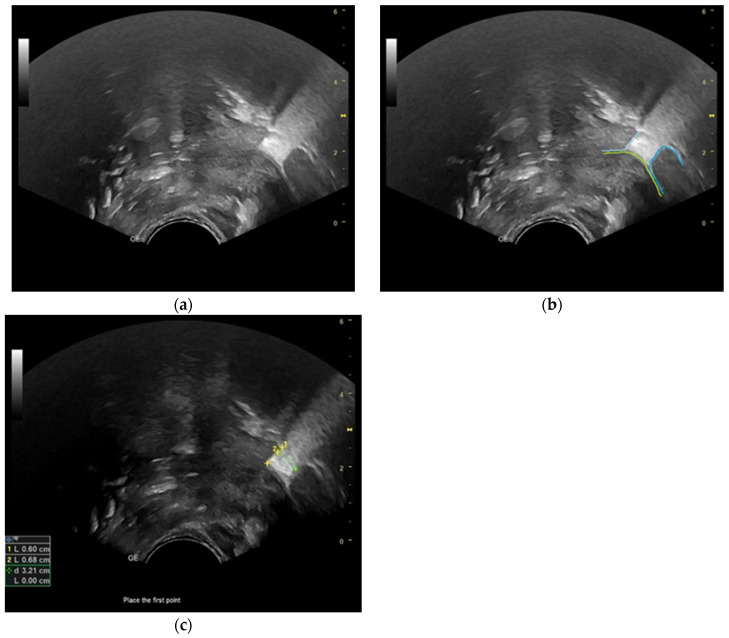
Ultrasonographic image of breastfeeding: (a) nipple view, tongue down; (b) nipple: dark blue line, HSPJ: light blue line, tongue: yellow line; (c) depth of intraoral space, cm: yellow line 1; nipple–HSPJ distance, cm: green line 2.
